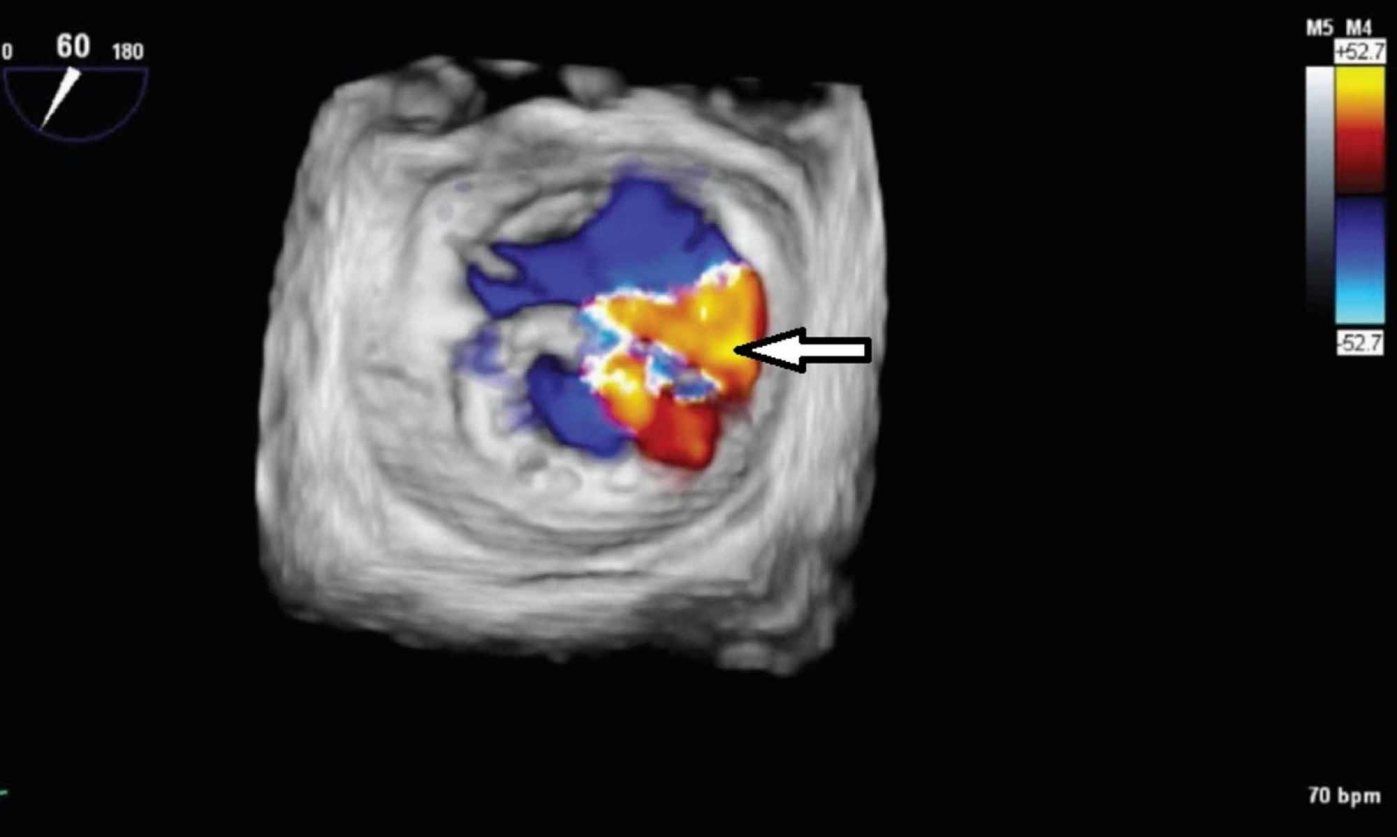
Severe mitral regurgitation (white arrow) and ring dehiscence noted on 3D Color Doppler TEE imaging of the mitral valve in en face view. TEE, transesophageal echocardiogram
